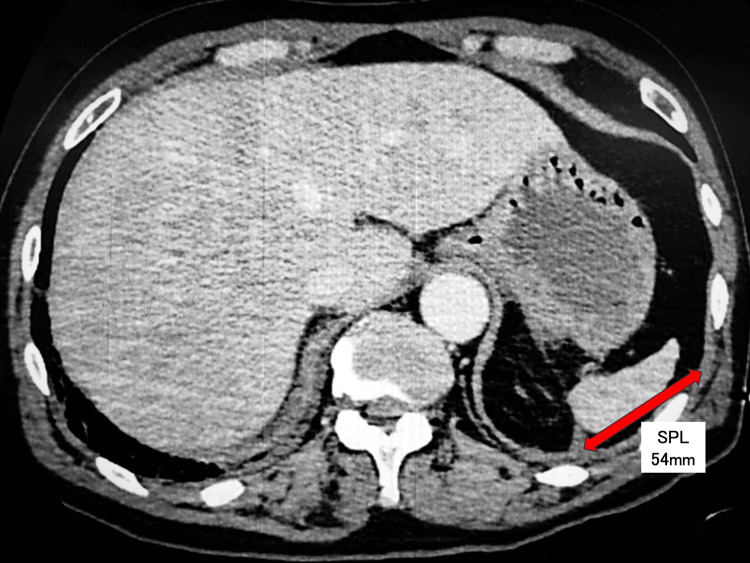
Abdominal computed tomography (CT) showing splenic atrophy. Axial view of the upper abdomen showing the spleen (SPL). The spleen is markedly small, with a maximum diameter of 54 mm (arrow). This finding indicates splenic atrophy.
